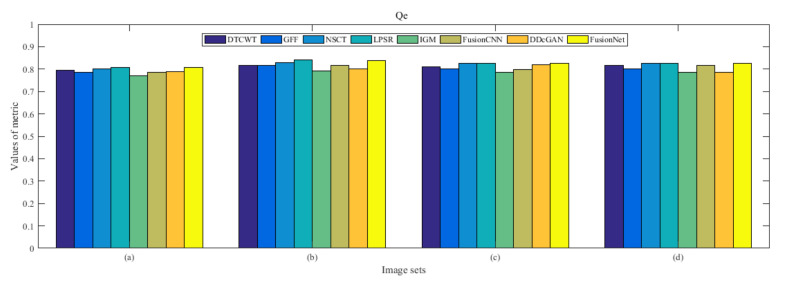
Values of $Q_{e}$ in the fused images (MRI-CBF): (a) is composed of eight images in Figure 42, (b) is composed of eight images in Figure 43, (c) is composed of eight images in Figure 44, (d) is composed of eight images in Figure 45.
